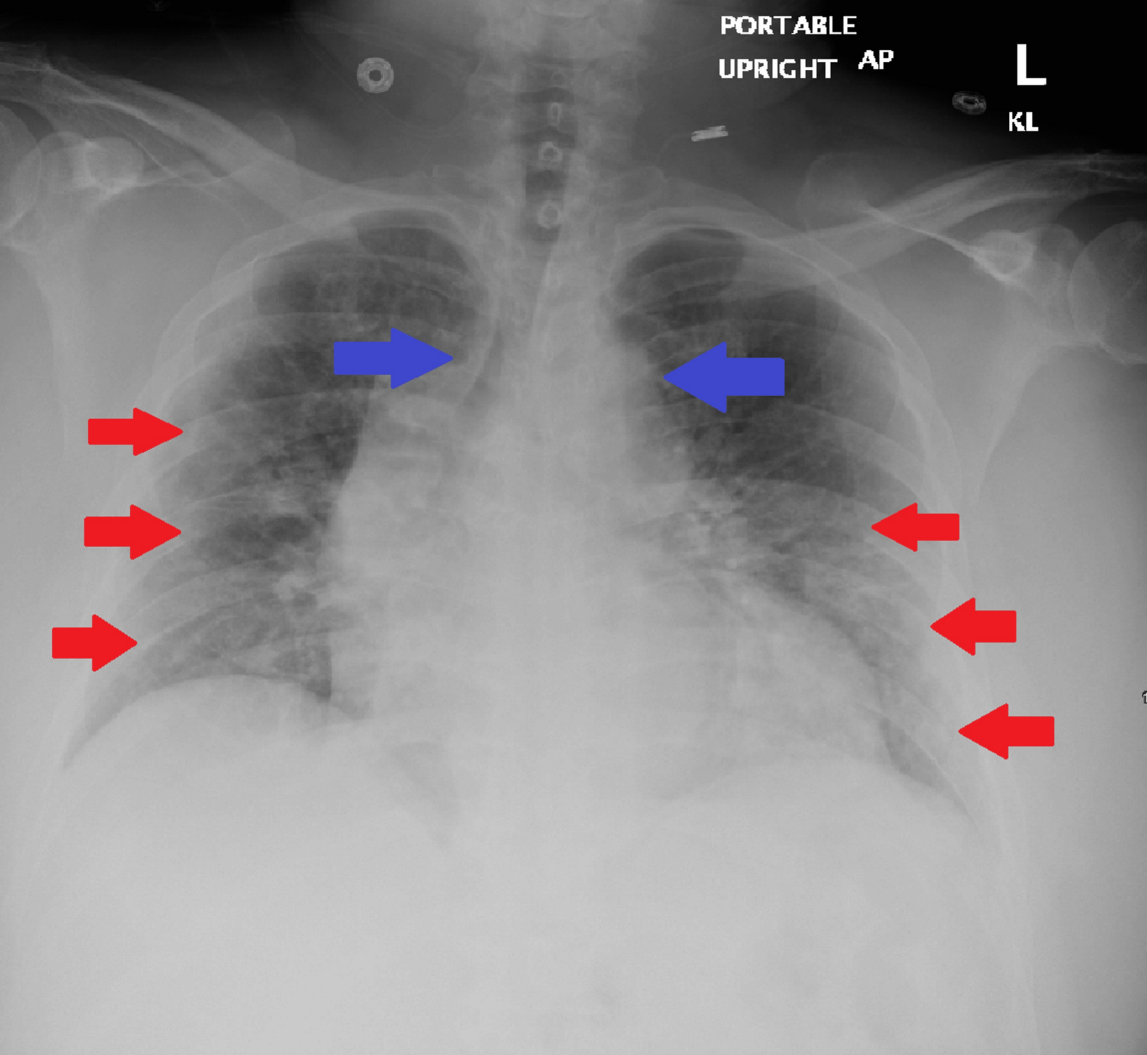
Chest X-ray AP: anteroposterior There is suboptimal inspiration with perihilar atelectasis and mild ground glass infiltrates which is worse on the left and highlighted by the red arrows which may represent suspected COVID-19 pneumonia. Of note, cardiomegaly is present with no large pulmonary effusions, edema, or pneumothorax. There is also mediastinal widening that is depicted by the blue arrows, but this may be due to vascular ectasia habitus, AP magnification, and/or possible other etiology. The patient's eosinophil sputum smear was also noted to be 6%
